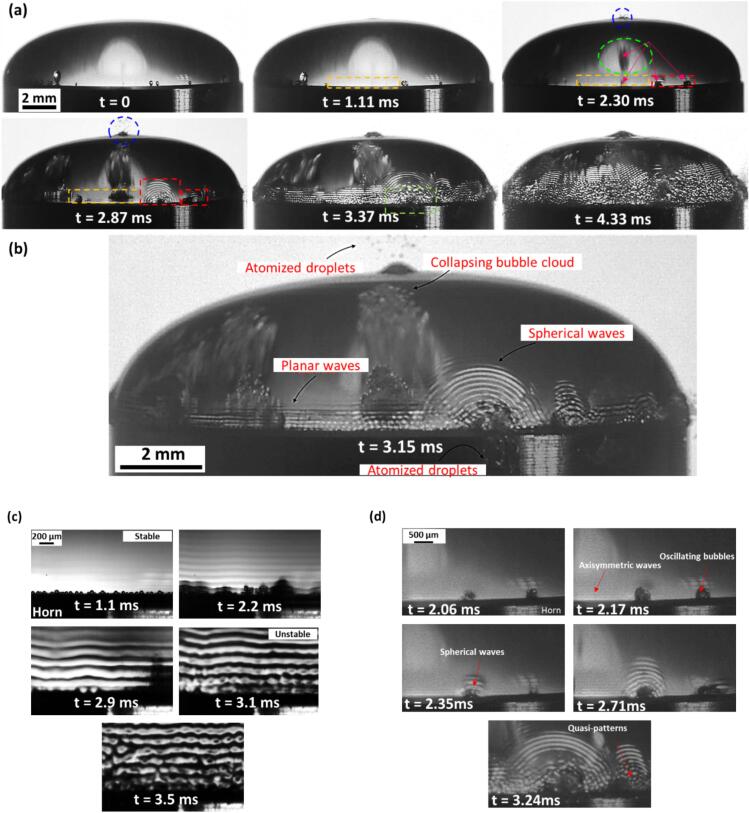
High-speed image sequence showing (a) initial stages of atomization, (b) a time instant showing formation of planar and spherical waves with collapsing bubble clouds and atomized droplets, (c) zoomed-in view of capillary wave evolution, and (d) interaction of planar (axisymmetric) waves with the spherical waves and their superimposition leading to surface wave instability, in a water droplet ultrasonically excited on a 14 mm horn tip.
